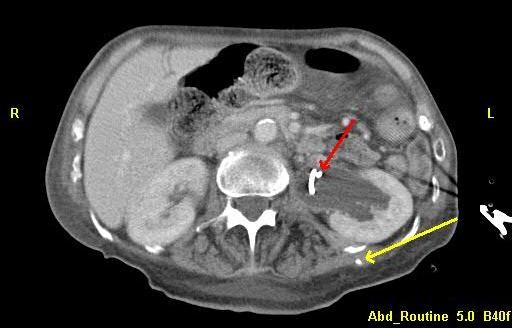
Computed tomography of the abdomen showing left-sided hydronephrosis with indwelling left ureteral stent (red arrow). Tip of the percutaneous drain is visualized in the left lateral back (yellow arrow).
